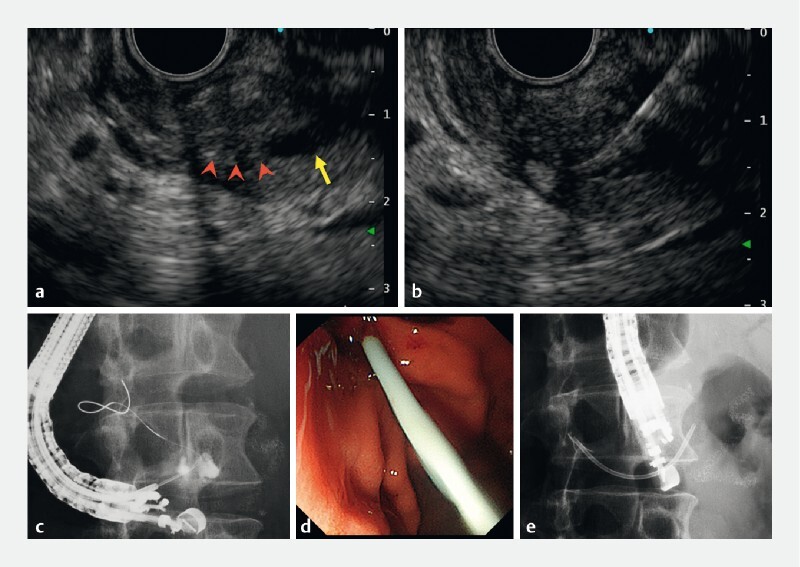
First attempt at endoscopic ultrasound (EUS)-guided re-pancreaticogastrostomy using a side-viewing echoendoscope.
